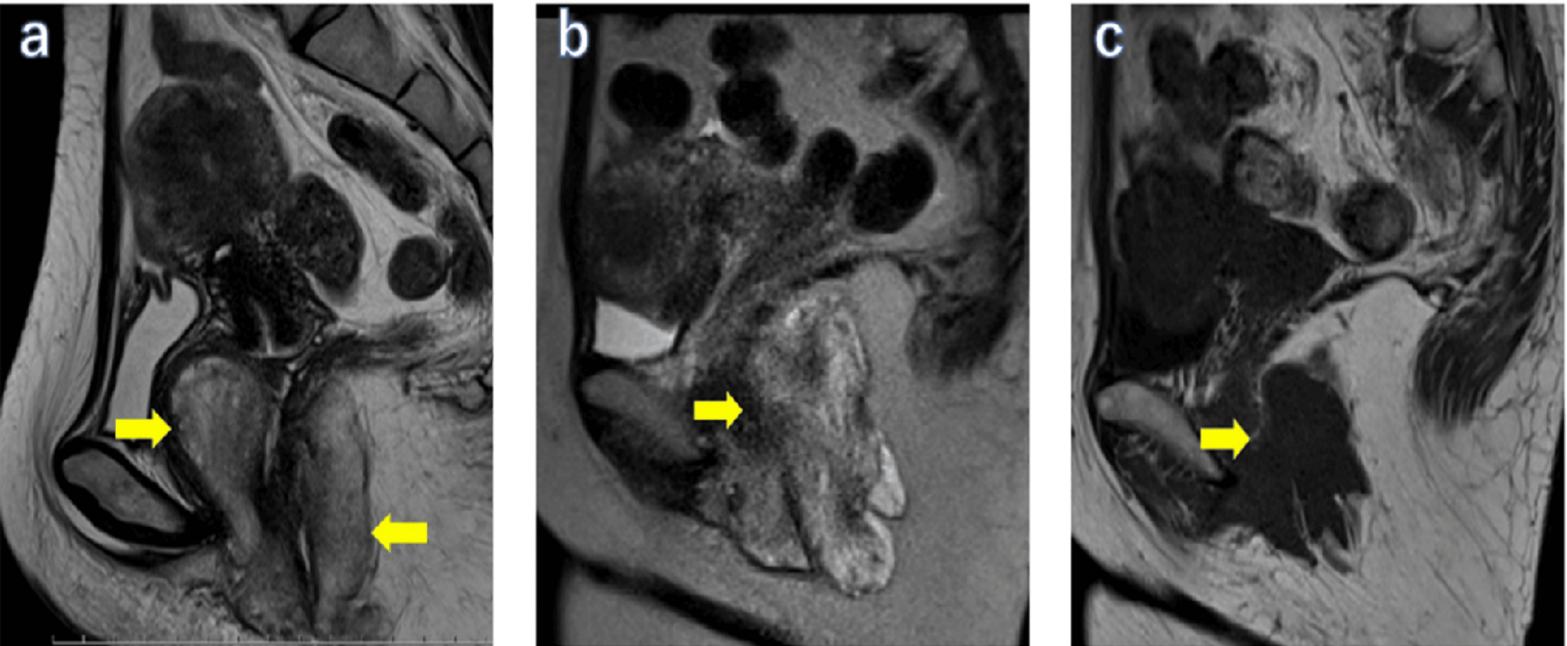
T2-weighted sagittal image of the patient's pelvic cavity MRI MRI: magnetic resonance imaging (a) Initial visit, (b) recurrence (11 months postoperatively), (c) the end of GnRH antagonist treatment (18 months postoperatively). A lobulated tumor with a diameter of 8 cm is seen from the left side of the vulva to the subvaginal mucosa, and the inside of the tumor shows a swirling and layering pattern (arrow) (a). A mass with a diameter of 8.6 cm and severe edematous changes similar to those seen at the initial examination are shown (b). Significantly reduced tumor of 4.6 cm after six months of relugolix administration (c)
